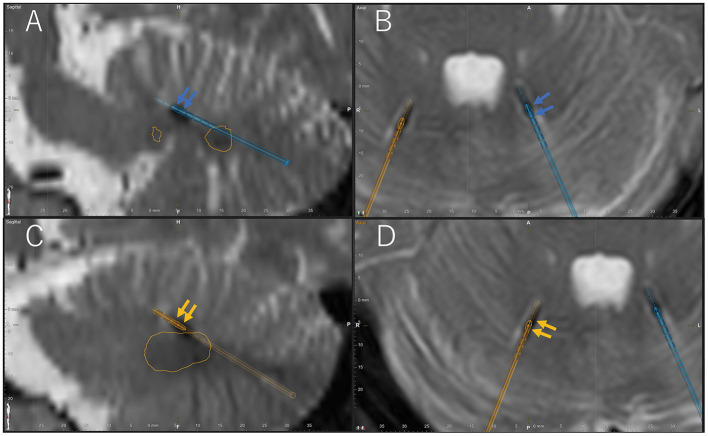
Location of active contacts of final stimulation settings confirmed by Brainlab Elements on the post-operative T2-weighted magnetic resonance imaging (MRI). Blue arrow indicates active cathode contacts of the left electrode (blue electrode) in the sagittal (A) and axial (B) postoperative T2-weighted MRI. Orange arrow indicates active cathode contacts of the right electrode (orange electrode) in the sagittal (C) and axial (D) postoperative T2-weighted MRI. Orange circle shows the bilateral dentate nucleus. A, anterior; P, posterior; H, head; F, foot; L, left; R, right.
